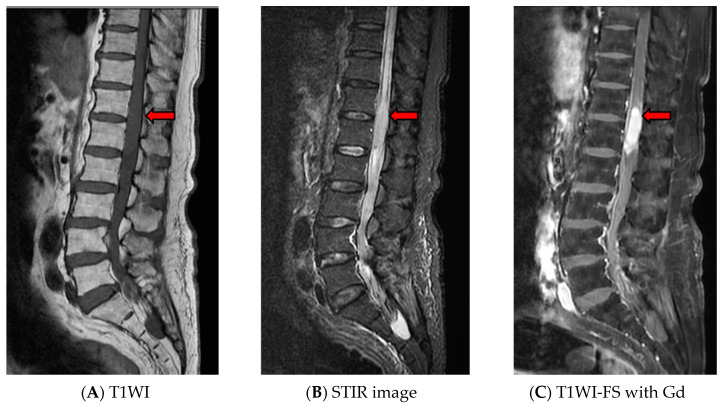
Magnetic resonance imaging of the lumbosacral spine revealed a 4.5 × 1.5 cm intramedullary expanding lesion at the T12-L1 level. The lesion exhibited isosignal intensity on the T1-weighted image (A) and hypersignal intensity on the Short tau inversion recovery (STIR) image (B) with homogeneous enhancement (C). Hypersignal intensity of the spinal cord above the lesion was also detected on the STIR image (B), which represented diffuse spinal cord edema.
